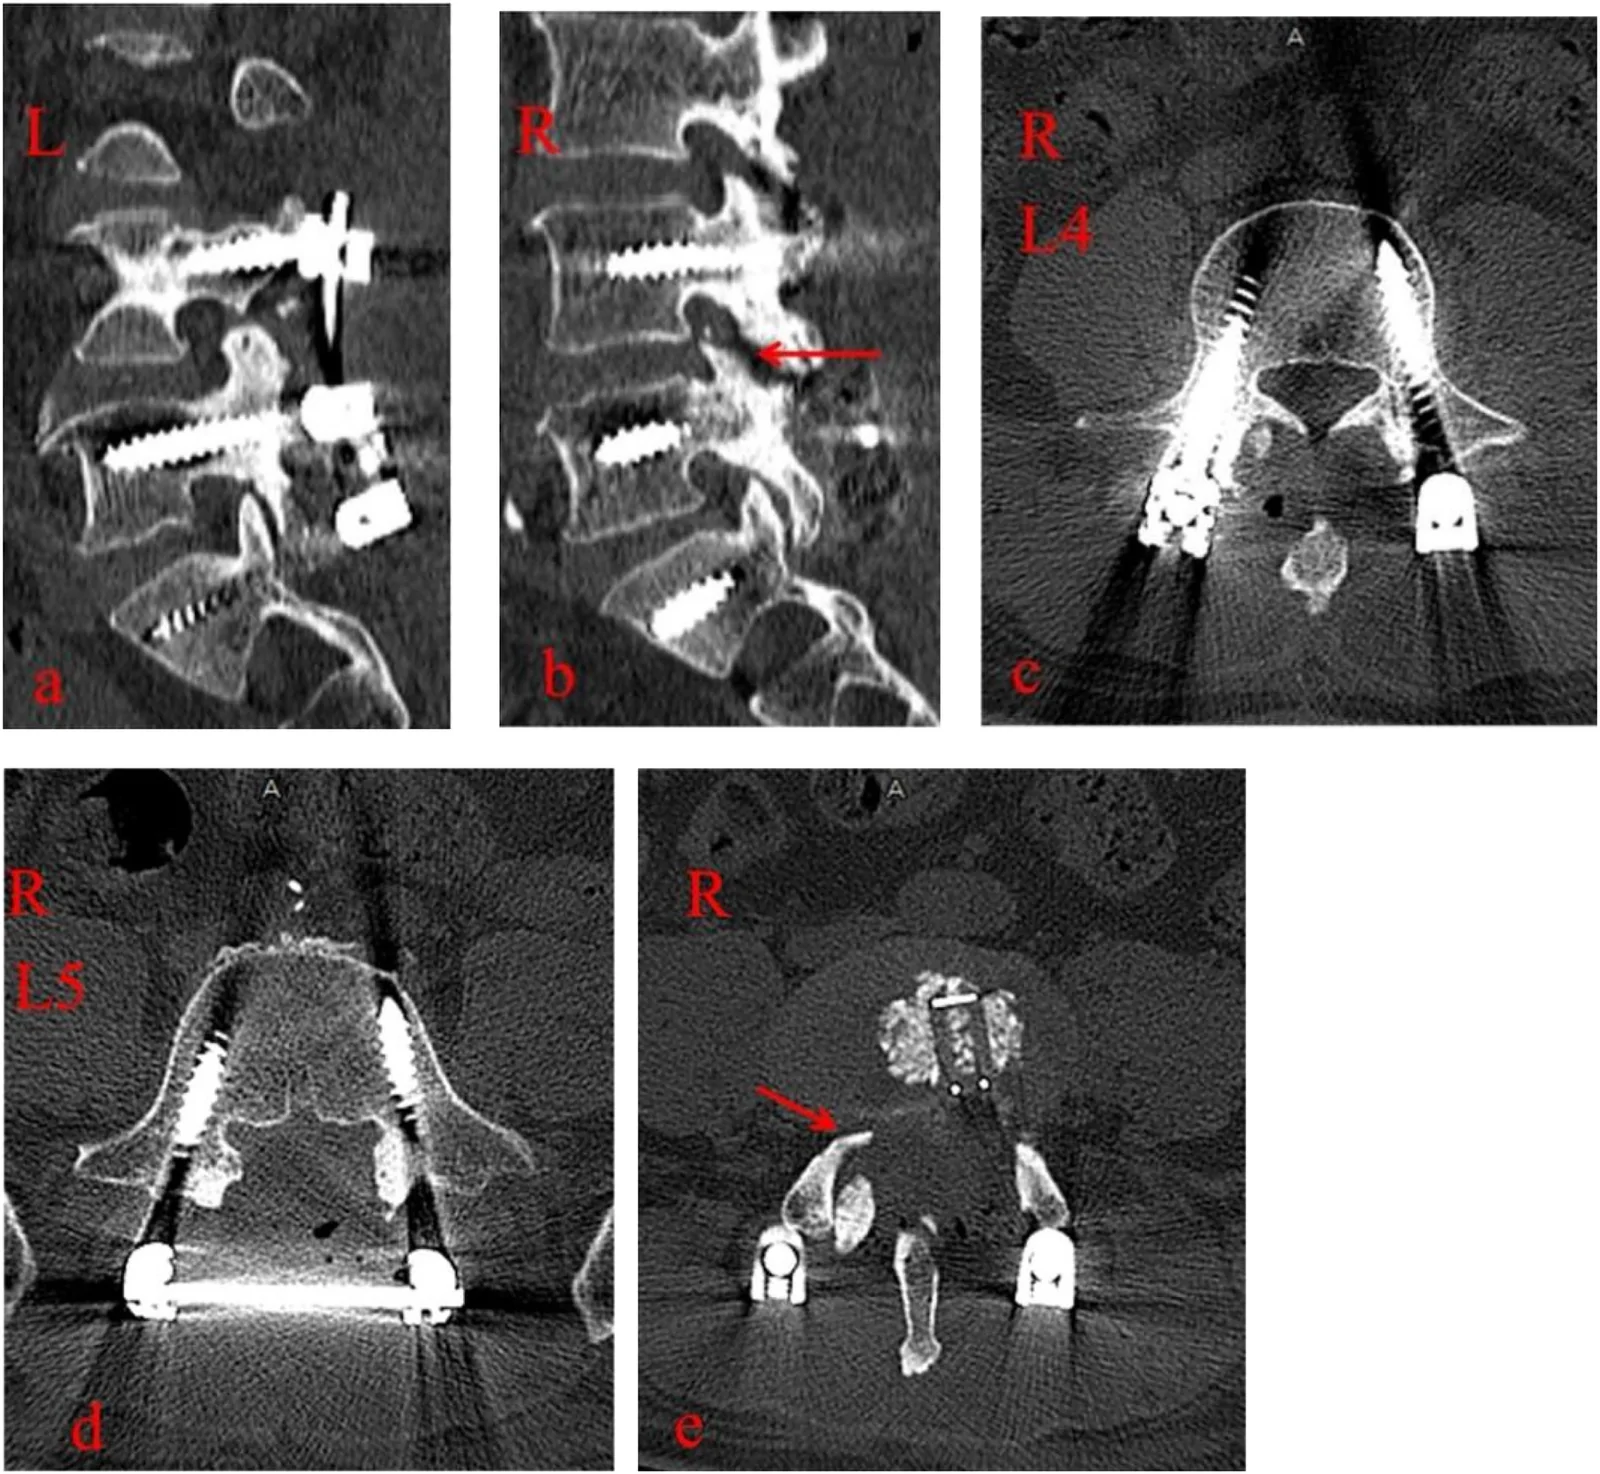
A postoperative CT demonstrated satisfactory lumbar spinal alignment, optimal positioning of the interbody cage, and pedicle screws (a–e). However, the residual right SAP reduced the width of the foramen on the right side (b,e).
